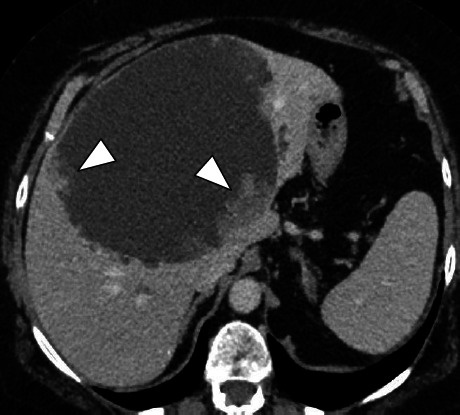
CT image in the axial plane at portal phase of enhancement. High-grade mucinous cystic tumor (formerly mucinous cystadenocarcinoma). Large cystic lesion in segment IV containing irregular, enhanced intracystic vegetations after contrast injection (arrowhead).
